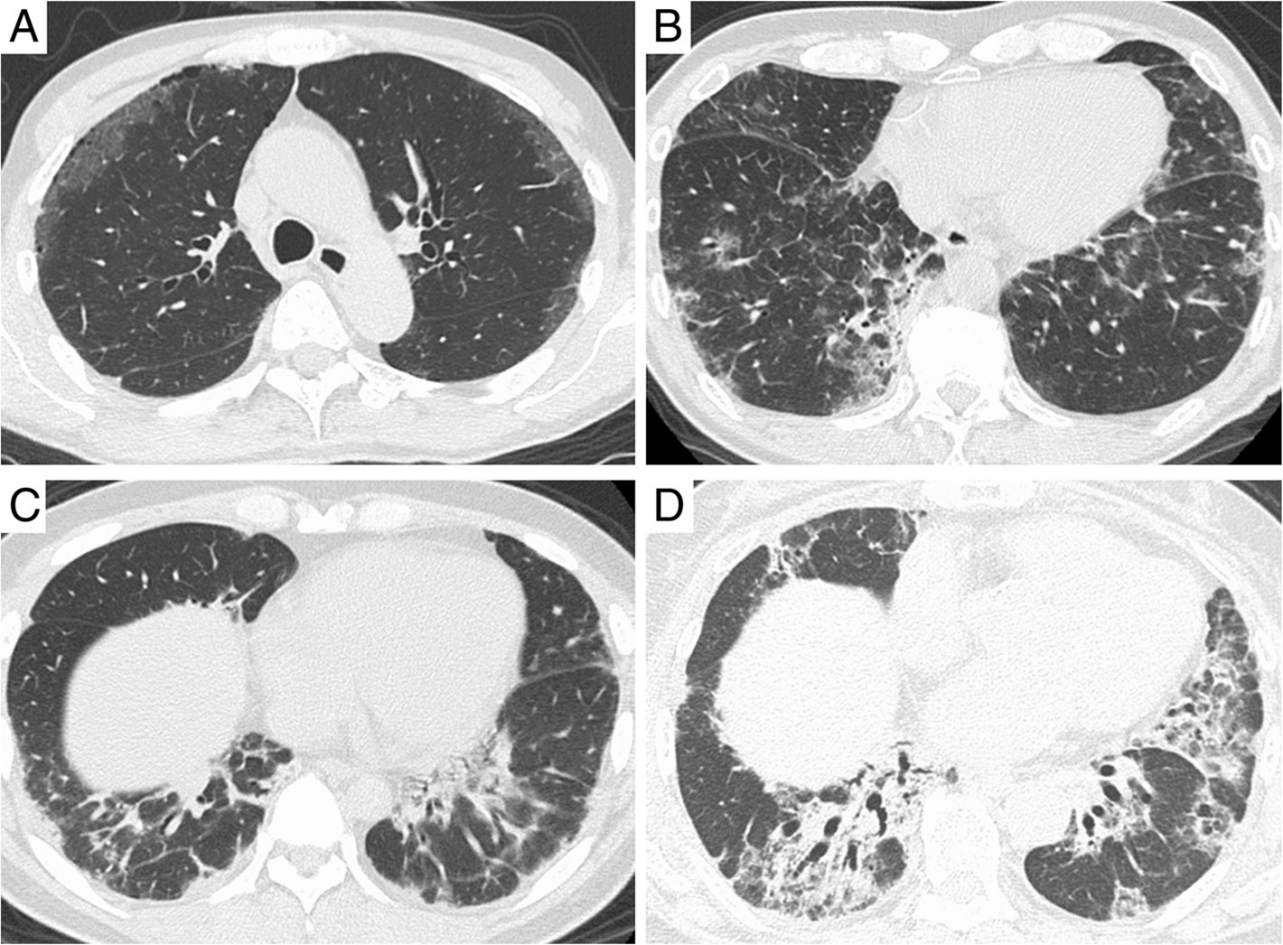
High-resolution computed tomography findings. Representative photographs of HRCT scans are presented. a and b initial HRCT scans of fatal cases positive for anti-MDA-5 antibody showing subpleural GGO. (C) HRCT scans of an anti-MDA-5 antibody-positive patient who survived, showing subpleural irregular linear opacity. (D) HRCT scans of an anti-MDA-5 antibody-negative patient showing peribronchovascular consolidation. Abbreviations: GGO, ground-glass opacity; HRCT, high-resolution computed tomography; MDA-5, anti-melanoma differentiation-associated gene 5
